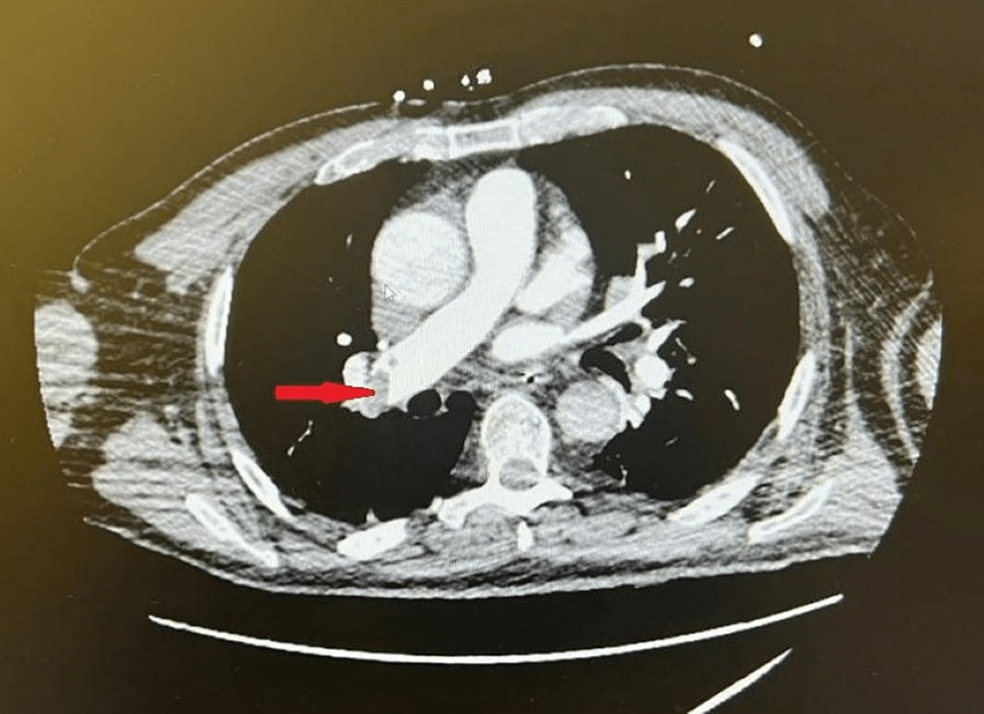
Axial image of the CT of the chest with intravenous contrast showing the right main pulmonary artery embolisms (red arrowhead).
